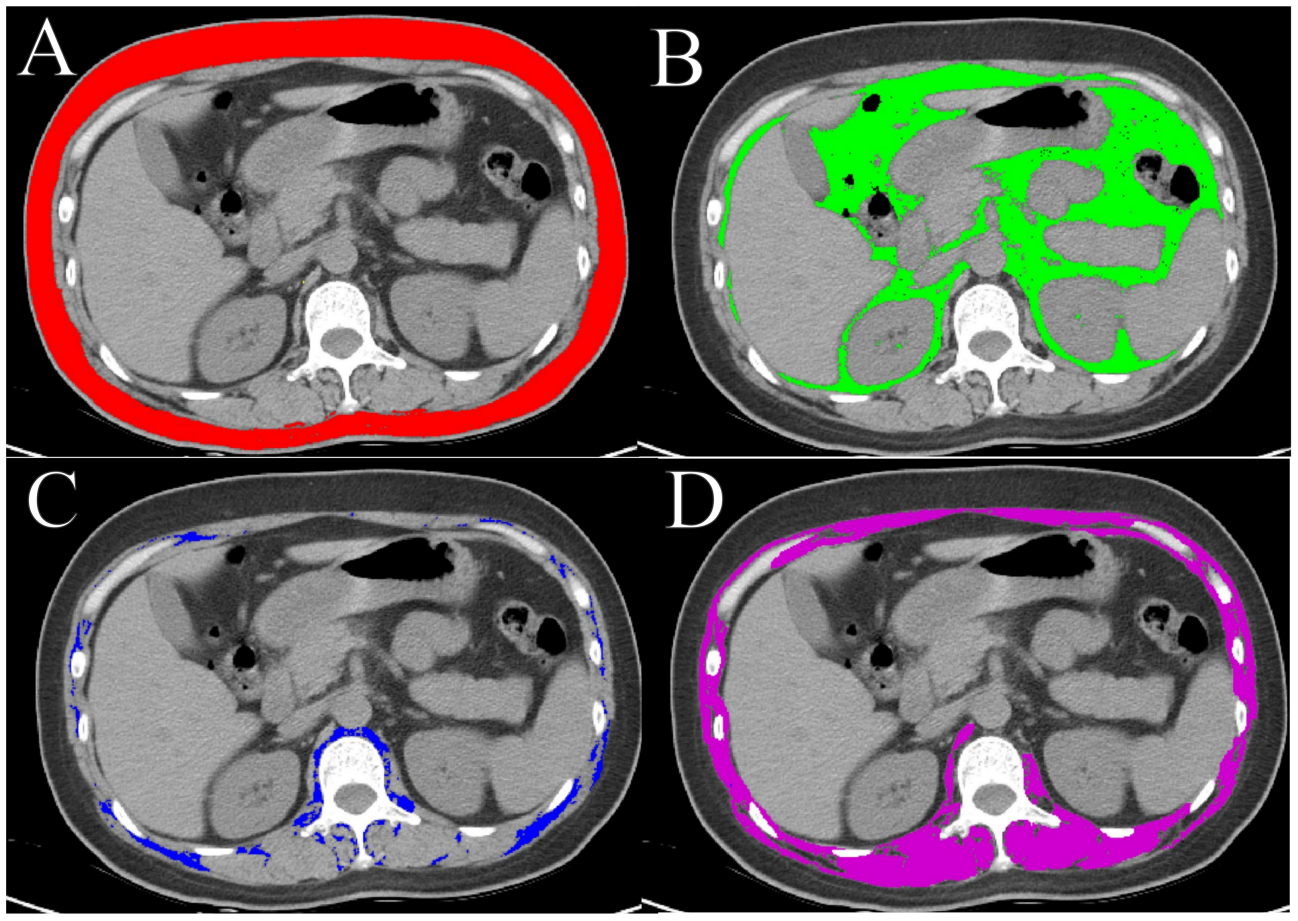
Body composition segmentation at the L1 level based on CT images. (A) Subcutaneous adipose tissue (SAT, red); (B) Visceral adipose tissue (VAT, green); (C) Intermuscular adipose tissue (IMAT, blue); (D) Skeletal muscle (SM, purple).
